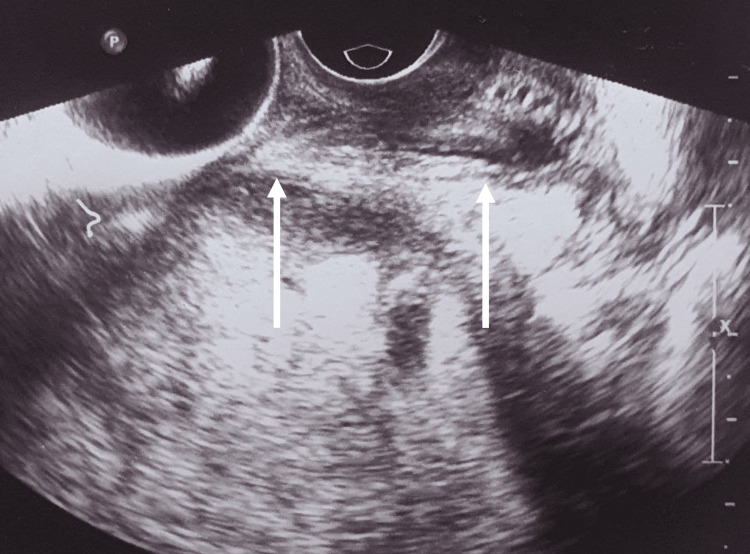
Transvaginal ultrasound showing the cervix (arrows) with closed internal and external os.
